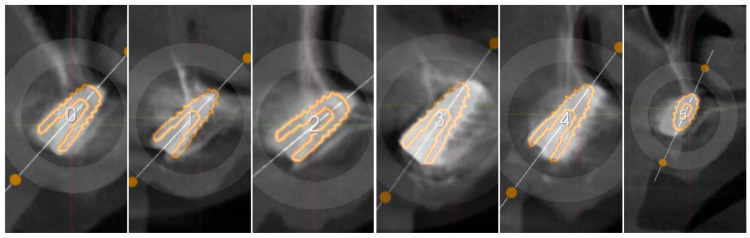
Super imposition of the digital plan and the Post-op CT scan. The numbers from 0 to 5 identify the implants placed.
